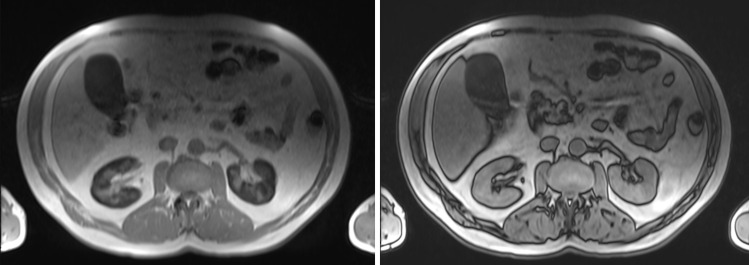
On MRI signal decrease is shown in the cortices of the kidneys on in-phase image comparing (a) to out-of-phase image (b).
